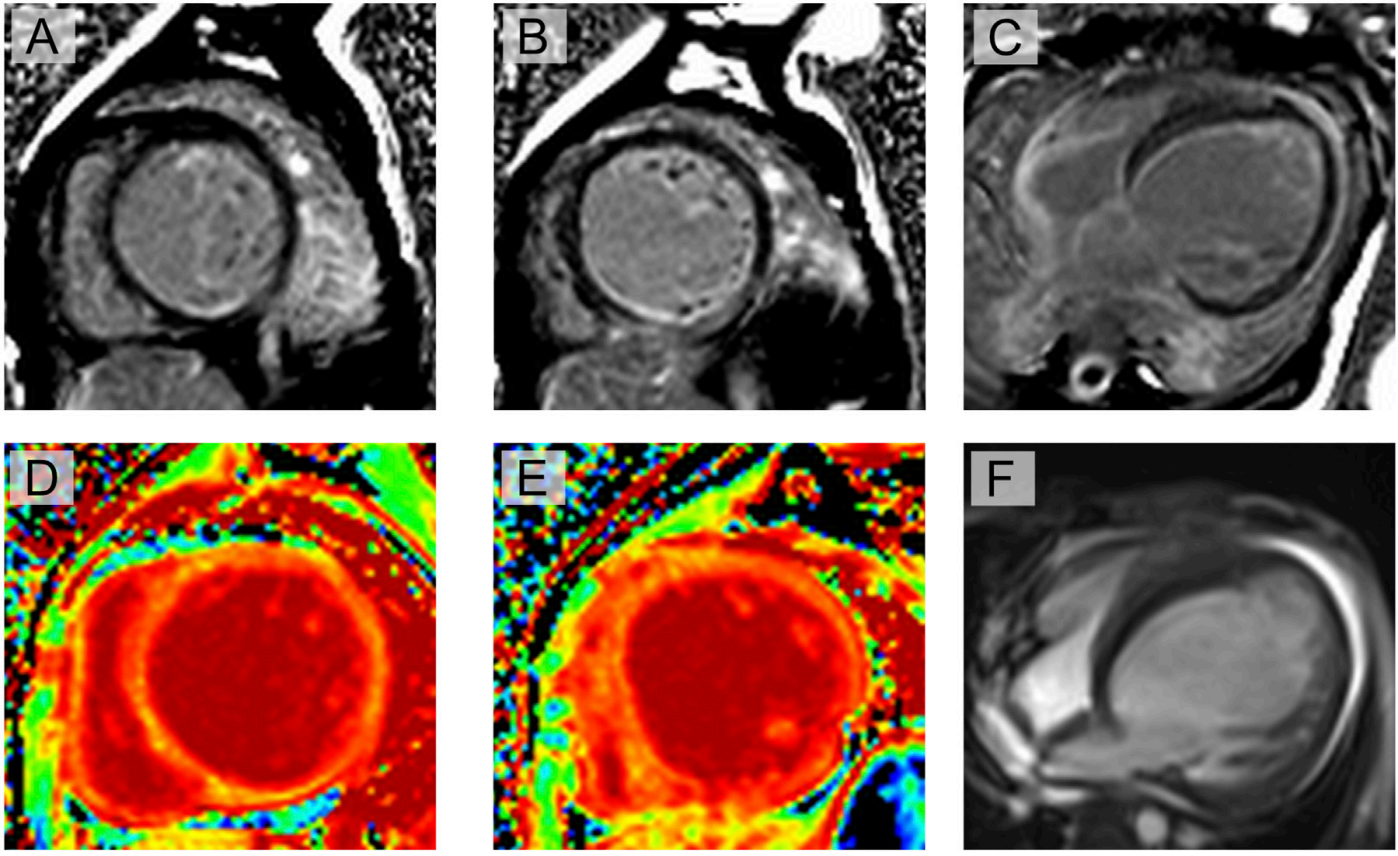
Cardiac magnetic resonance imaging evaluation of the proband: (A–C) late gadolinium enhancement shows myocardial fibrosis at multiple locations, especially in the muscle layer of the interventricular septal wall and subendocardial layer of the left ventricle (yellow arrow); (D, E) T1 mapping shows significantly increased extracellular volume; (F) cine imaging shows enlarged left ventricle, significantly reduced left-ventricular motion, and reduced systolic function (ejection fraction = 21%). Hypertrabecularization was also observed in the free wall and apex of the left ventricle.
